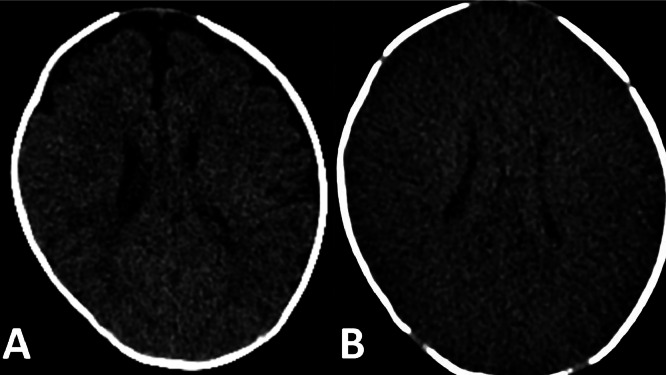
(A) Axial computed tomography (CT) of the head without contrast on day 1 which was read as normal at the outside hospital. (B) Axial CT of the head without contrast on day 2 after clinical changes were noted with diffuse loss of grey-white differentiation and sulcal effacement.
